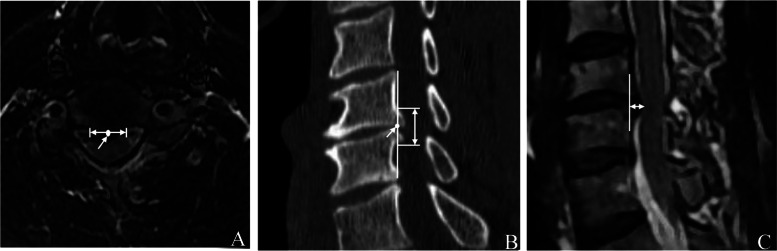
The measurement method of the width of the disc-osteophyte complex on MRI,The center point of the width was the center of the transverse position (A); The measurement method of the height of the disc-osteophyte complex on CT, The center point of the height was regarded as the center of the sagittal lesion position (B); The measurement method of the depth of the disc-osteophyte complex on MRI (C); (white double arrow)
